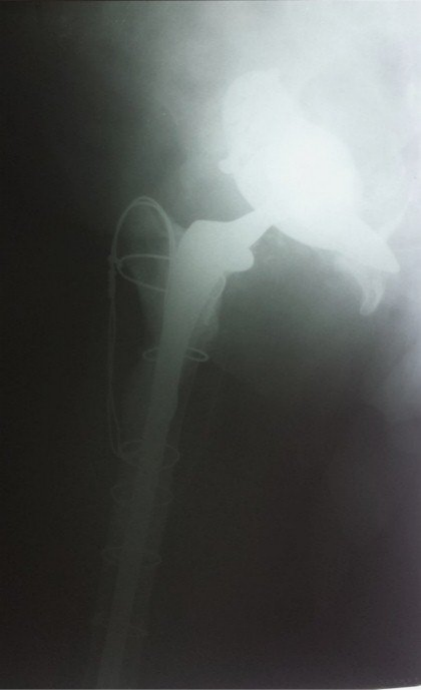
Radiographie postopératoire, prothése totale de hanche avec tige fémoeale longue et armature de Burch-Schneider
